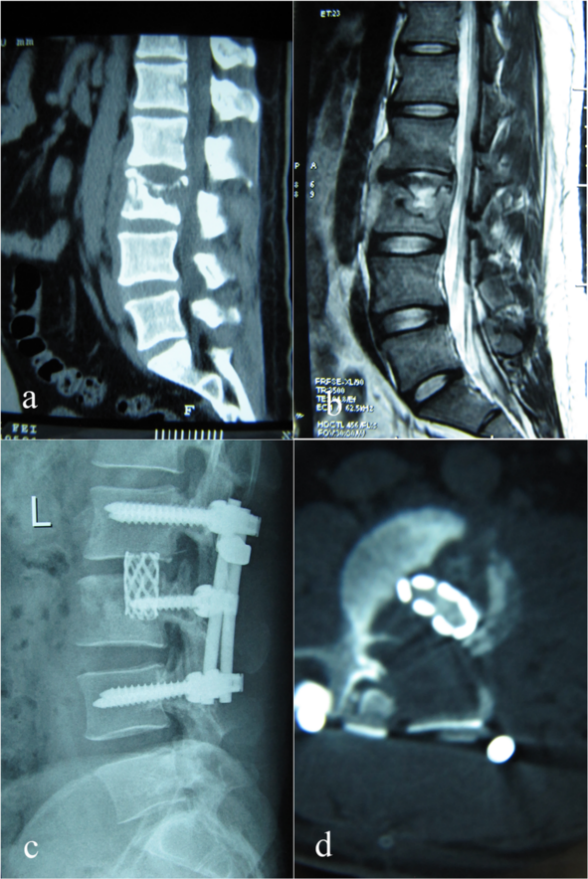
Case number 17. Preoperative image evaluation. a Computed tomography (CT) and b magnetic resonance imagery (MRI) showing L3 involvement. c, d At final follow-up, the internal fixation was in good shape and interbody fusion had been obtained, without signs of tuberculosis recurrence
